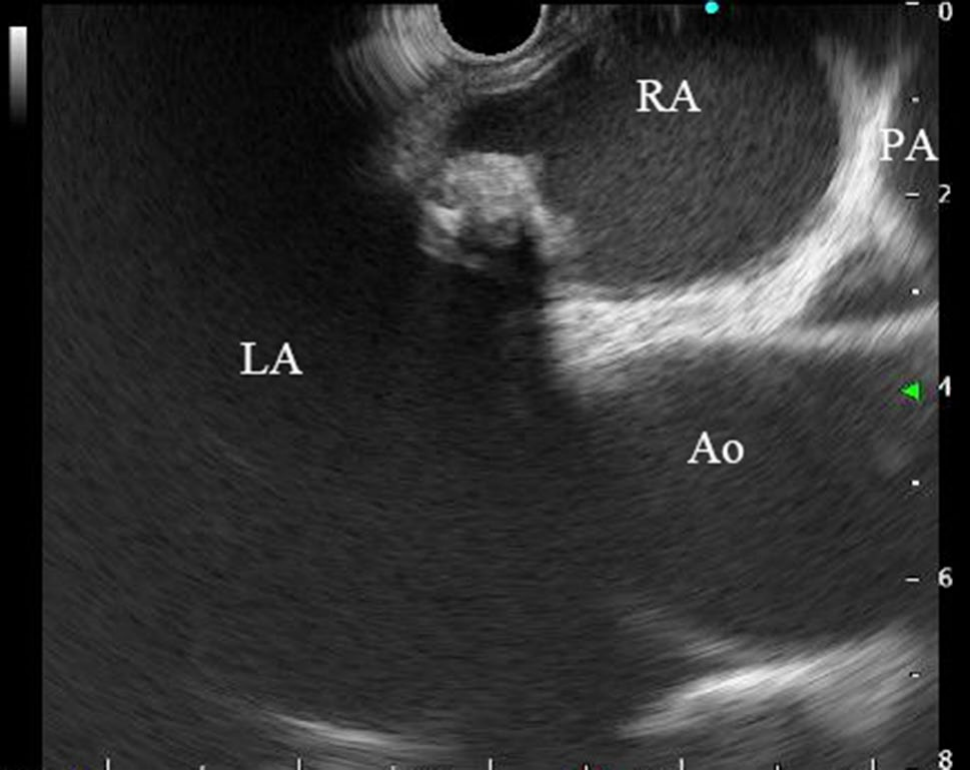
In endoscopic ultrasound, observation of the mediastinum revealed a lesion measuring 15 mm × 9 mm with heterogeneous echoic pattern and partly calcified pattern at the left atrial wall. *LA* left atrium, *RA* right atrium, *Ao* aorta, *PA* pulmonary artery
